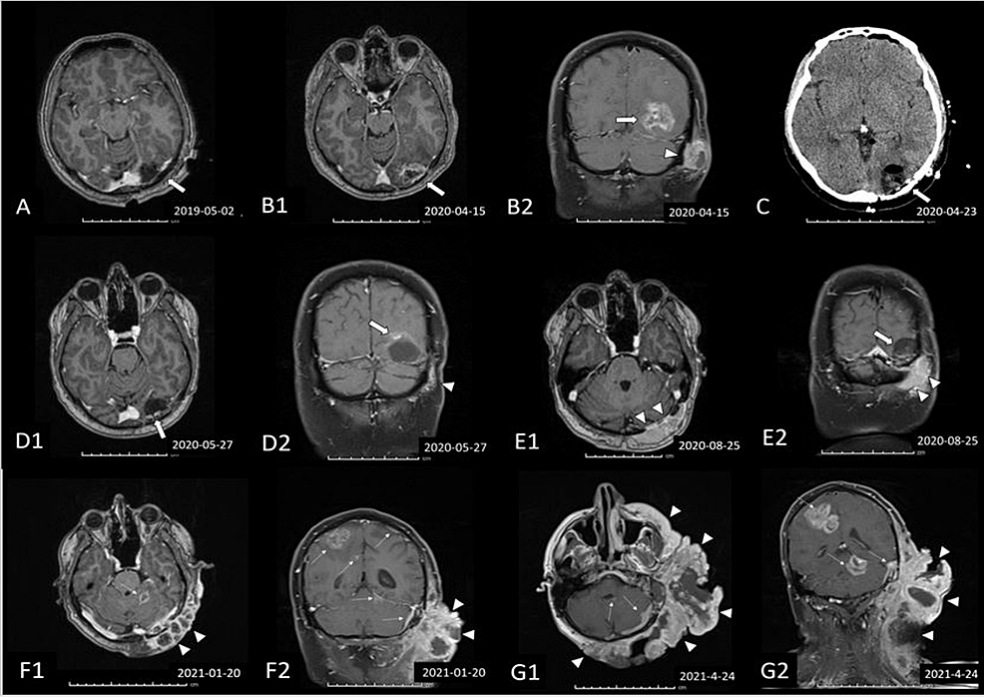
Postoperative findings after GBM (WHO grade IV) resection in the left occipital lobe (not shown). T1-weighted, gadolinium-enhanced axial and coronal MRI scans and axial CT image. (A) axial T1-weighted scan after the first surgery demonstrating a small residual tumor posterior to the surgical cavity. (B) Axial T1-weighted scan obtained approximately one year later showing residual tumor and a new scalp mass. (C) Axial CT image after the second surgery changes without residual tumor. (D) Following re-resection of the tumor, a local breakthrough through the extracranial soft tissue was observed. (E1-2 and F1-2) Axial and coronal T1-weighted scans showing significant enlargement of the extracranial mass. (G1-2) Nine months after the second surgery, new multiple contrast-enhanced masses with cystic degeneration were seen in the cerebral and cerebellar hemispheres, showing progressive disease with intra and extracranial heterogeneously enhancing masses. Arrow, GBM: arrowheads, local progression of the GBM in the extracranial soft tissue; thin arrows: intracranial metastasis GBM: glioblastoma multiforme; WHO: World Health Organization; MRI: magnetic resonance imaging; CT: computerized tomography
